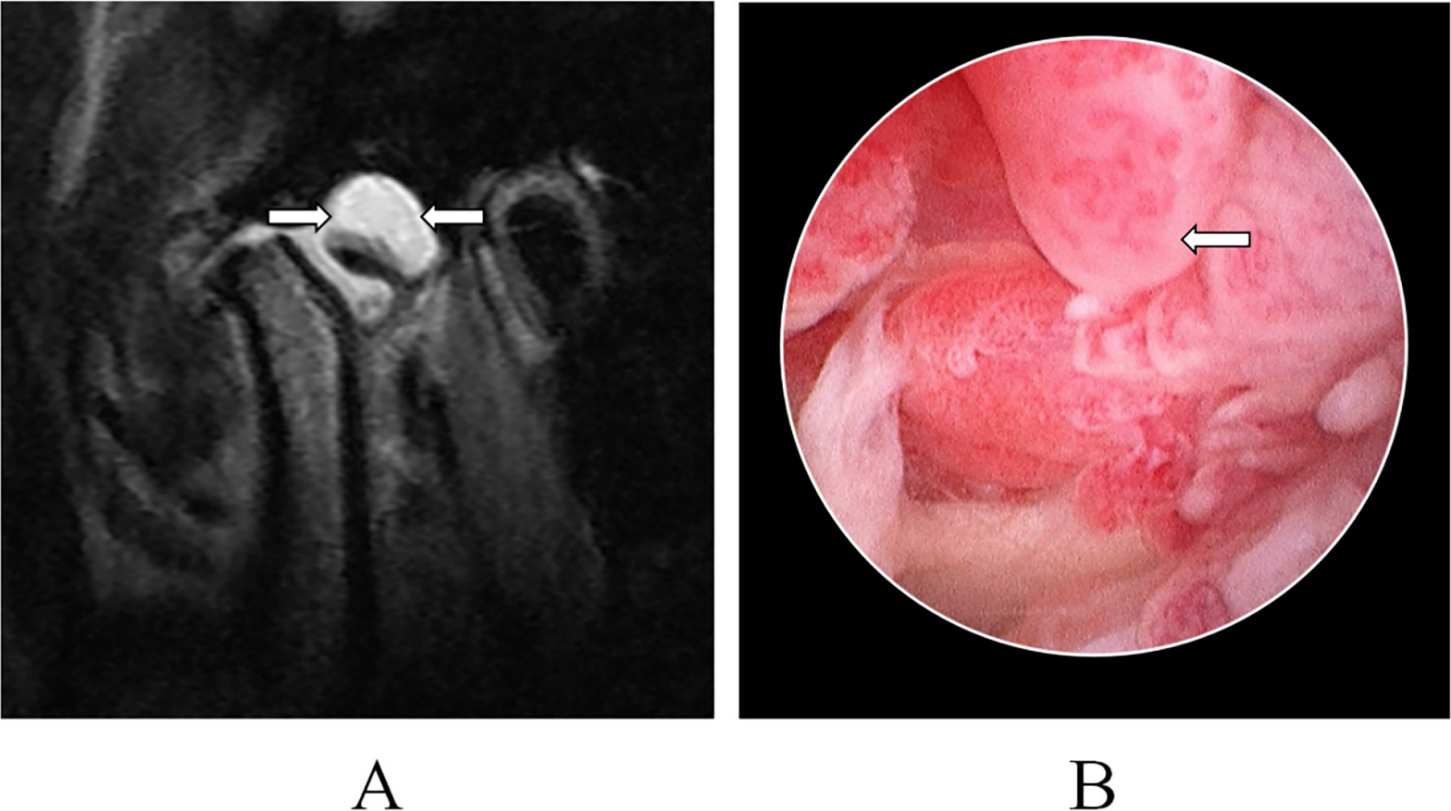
Synovitis of temporomandibular joint: hyperintense synovial fluid was uniformity and moderate signal mass (arrow) was observed on a T2 sequence of MRI, A; No loose body was detected during arthroscopic surgery, B.
